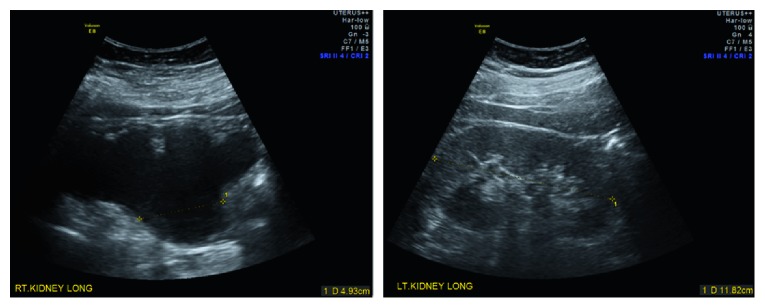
Kidney ultrasound scan showed severe right hydronephrosis with parenchymal thinning and normal left kidney.
